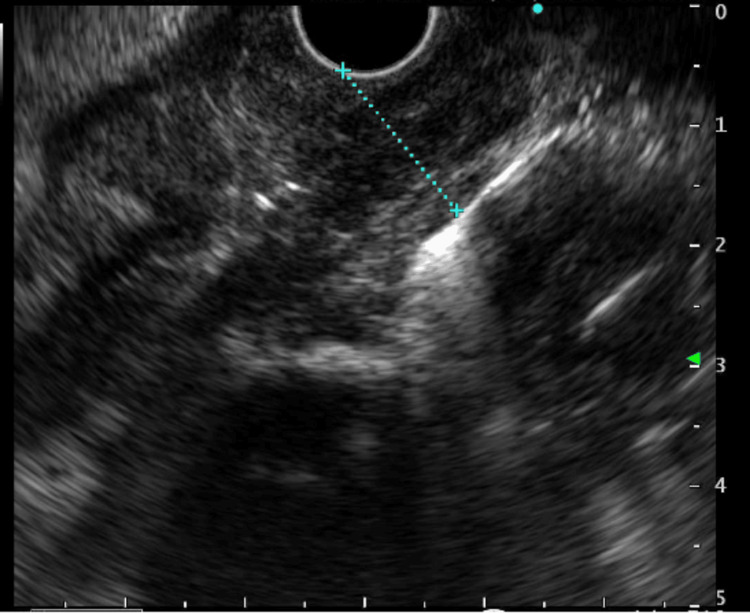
Endoscopic ultrasound depicting a heterogenous mass, as measured by the blue line, is seen invading past the muscularis propria.
